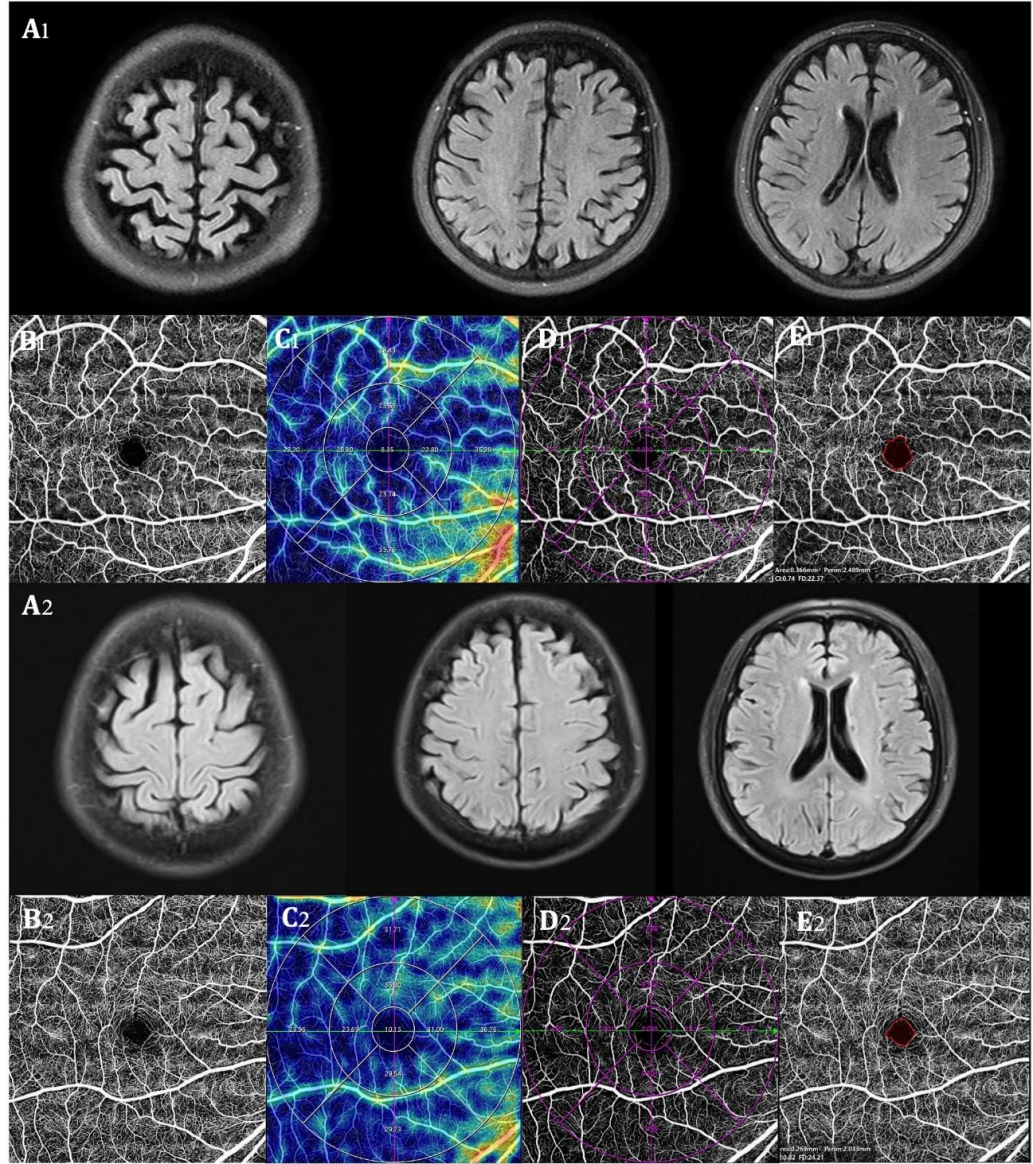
Comparisons of OCTA parameters between an AD patient and a healthy control. A 76-year-old female was admitted to the hospital due to “memory loss for one week”. The baseline brain MRI T2-FLAIR sequence showed an obvious cortical atrophy in her brain (A1). Genetic and CSF testing results supported AD diagnosis because she had ApoE e4 and A+T+. Compared with the healthy control (had no cognitive impairment and no brain atrophy on brain MRI T2-FLAIR, A2), the AD patient showed a decrease in retinal VD (B1 *vs.* B2), the SRCP VD (C1 *vs.* C2) and perfusion area (D1 *vs.* D2), but an increase in FAZ area (E1 *vs*. E2) on OCTA. A+: soluble Aβ 42/40 ratio decrease in CSF; T+: increase p-Tau181 protein content in CSF. MRI, magnetic resonance imaging; CSF, cerebrospinal fluid; AD, Alzheimer's disease; OCTA, optical coherence tomography angiography; SRCP, superficial retinal capillary plexus; VD, vessel density; FAZ, fovea avascular zone.
